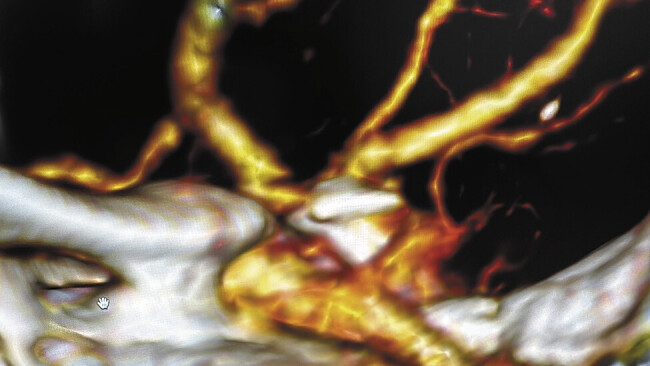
CTA reconstruction showing blood-blister like aneurysm located in the C7 segment of left ICA.
